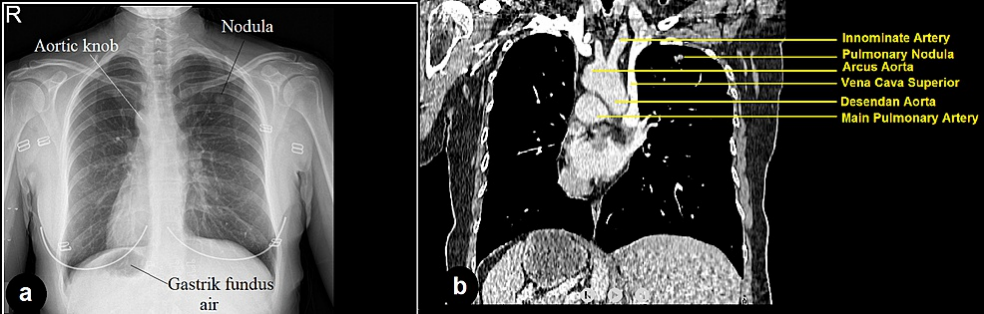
Chest X-ray showing situs inversus totalis and a solitary pulmonary nodule located in left upper zone; b: Thorax CT shows mirror image of vascular structures.
